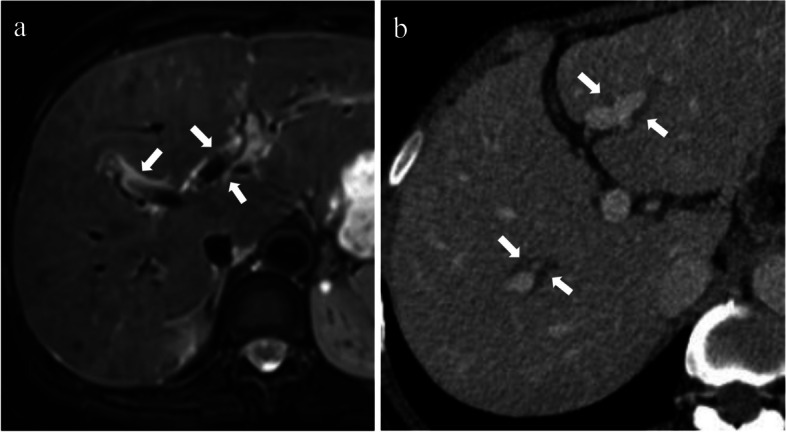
Periportal edema of PBC. a T2WI image of a 54-year-old female with PBC shows periportal hyperintensity (arrows). b Contrast-enhanced CT of a 53-year-old female with PBC shows periportal low density (arrows)
